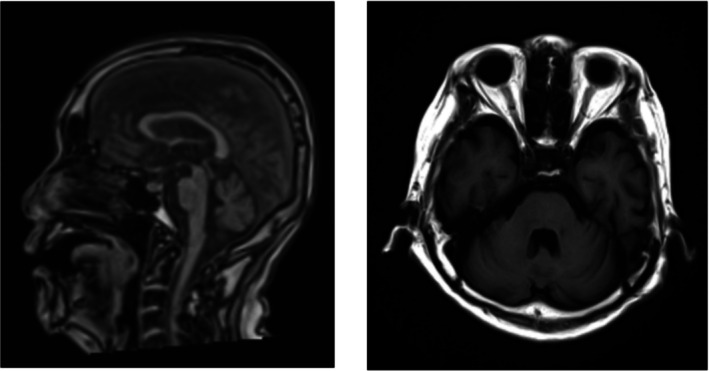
Brain magnetic resonance imaging showing no structural abnormalities of the hypothalamic–pituitary region. No evidence of pituitary or hypothalamic lesions was identified, suggesting the absence of a structural hypothalamic–pituitary cause.
